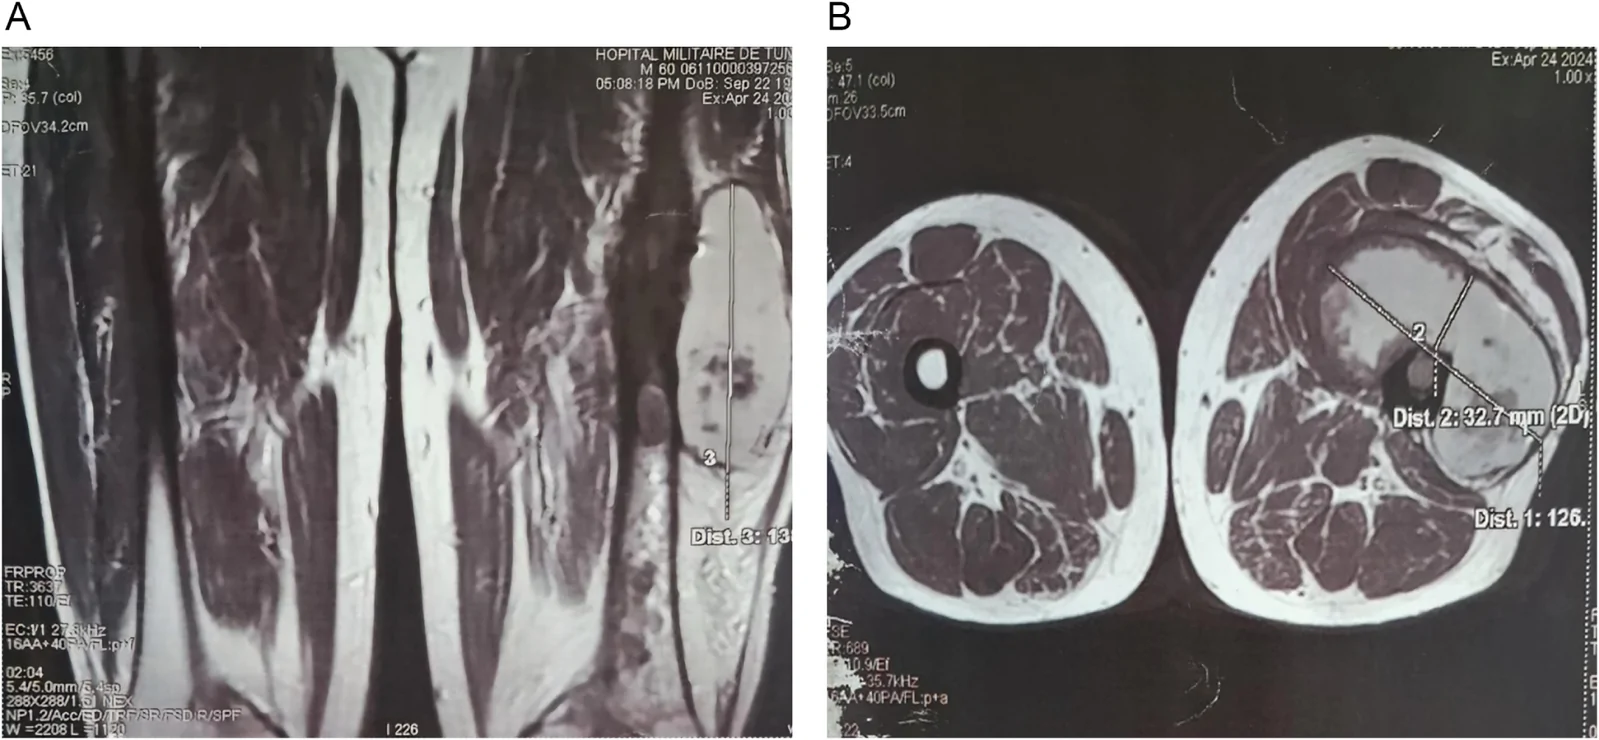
(A and B) Magnetic resonance imaging of the left thigh in sagittal (Fig. 2A) and axial views (Fig. 2B) showing the collection.
